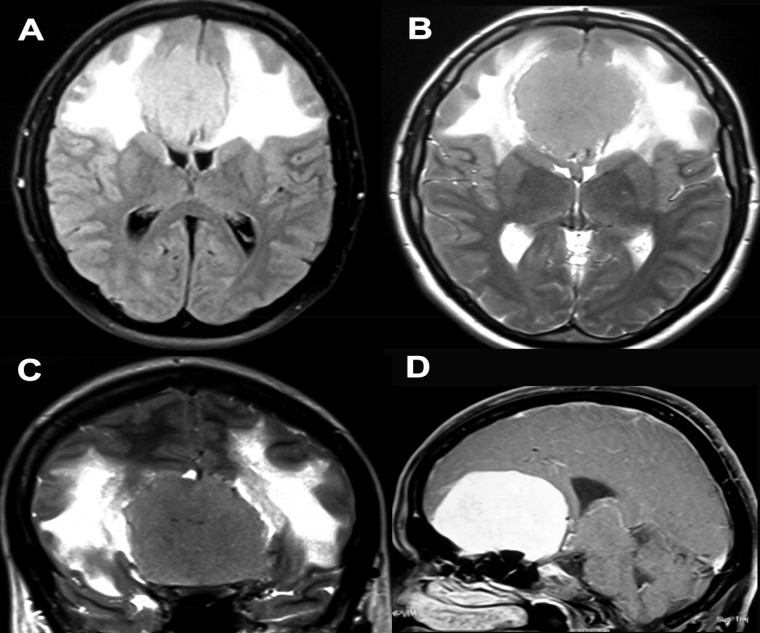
Brain magnetic resonance imaging (MRI) of the patient: Axial FLAIR and T2-weighted brain MRI with hypersignal lesion in midline of frontal area (A, B), coronal FLAIR demonstrating the mass with intense edema (C), and T1-weighted sagittal MRI indicating avidly enhancement of the mass after contrast administration
